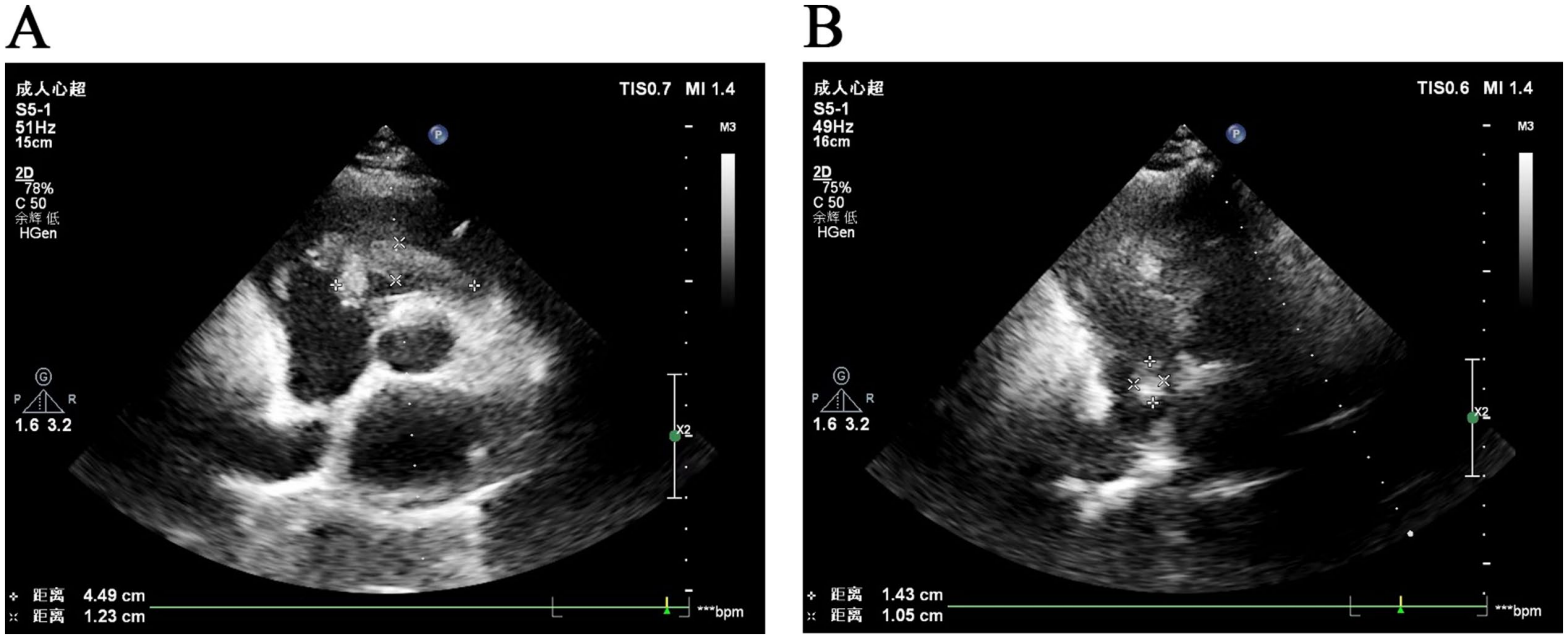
Ultrasonographic images of intravascular leiomyomatosis. (A) Ultrasonographic image shows a mixed-echo mass located on the tricuspid valve. (B) Ultrasonographic image shows a fibrotic mass near the right atrial septum.
